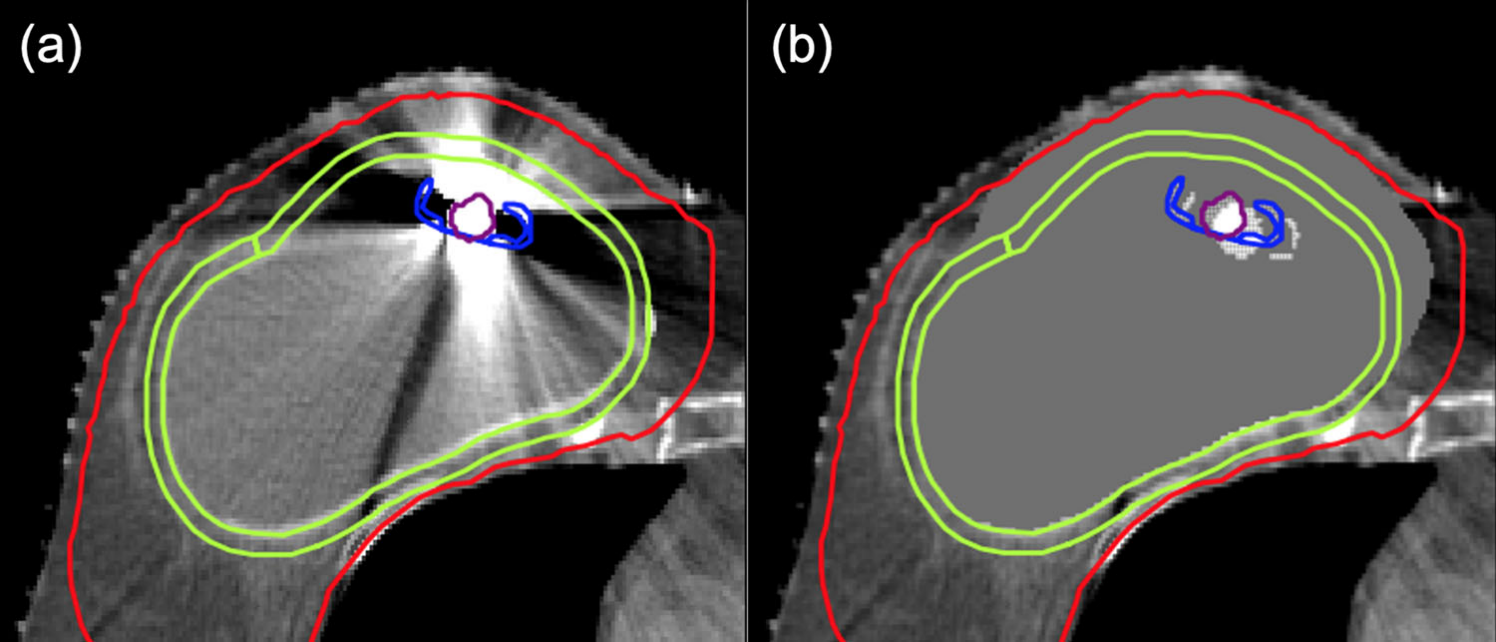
Computed Tomography (CT) slice of a representative patient before panel (a) and after panel (b) density overrides of the metal port and the tissue surrounding it. The maroon and blue contours are the magnetic core and titanium shell of the metal port, respectively. The area enclosed by the green contour is the region of interest (ROI), defined as a 5‐mm expansion around the saline implant. The red contour is the clinically defined planning target volume (PTV). The silhouette of the metal port in panel (b) demonstrates the artificially shifted metal port used for modeling internal port errors
